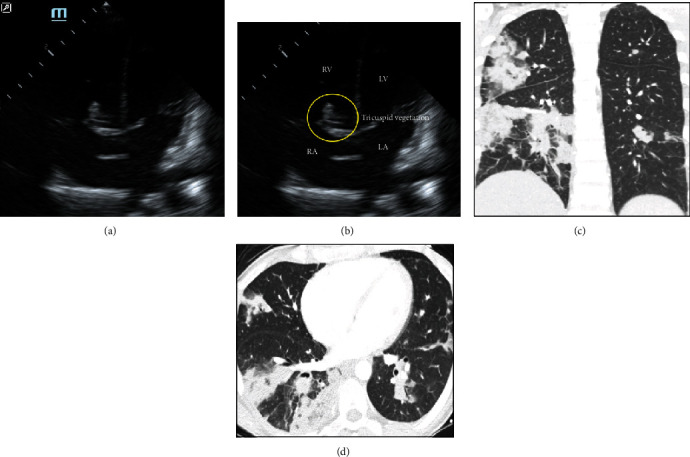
(a, b) Representative images from the bedside echocardiogram (apical four-chamber view) demonstrating tricuspid vegetations (circle). (c, d) Representative images from the CT angiogram (coronal and axial images, respectively) revealing the right-lower-lobe wedge infarct.
